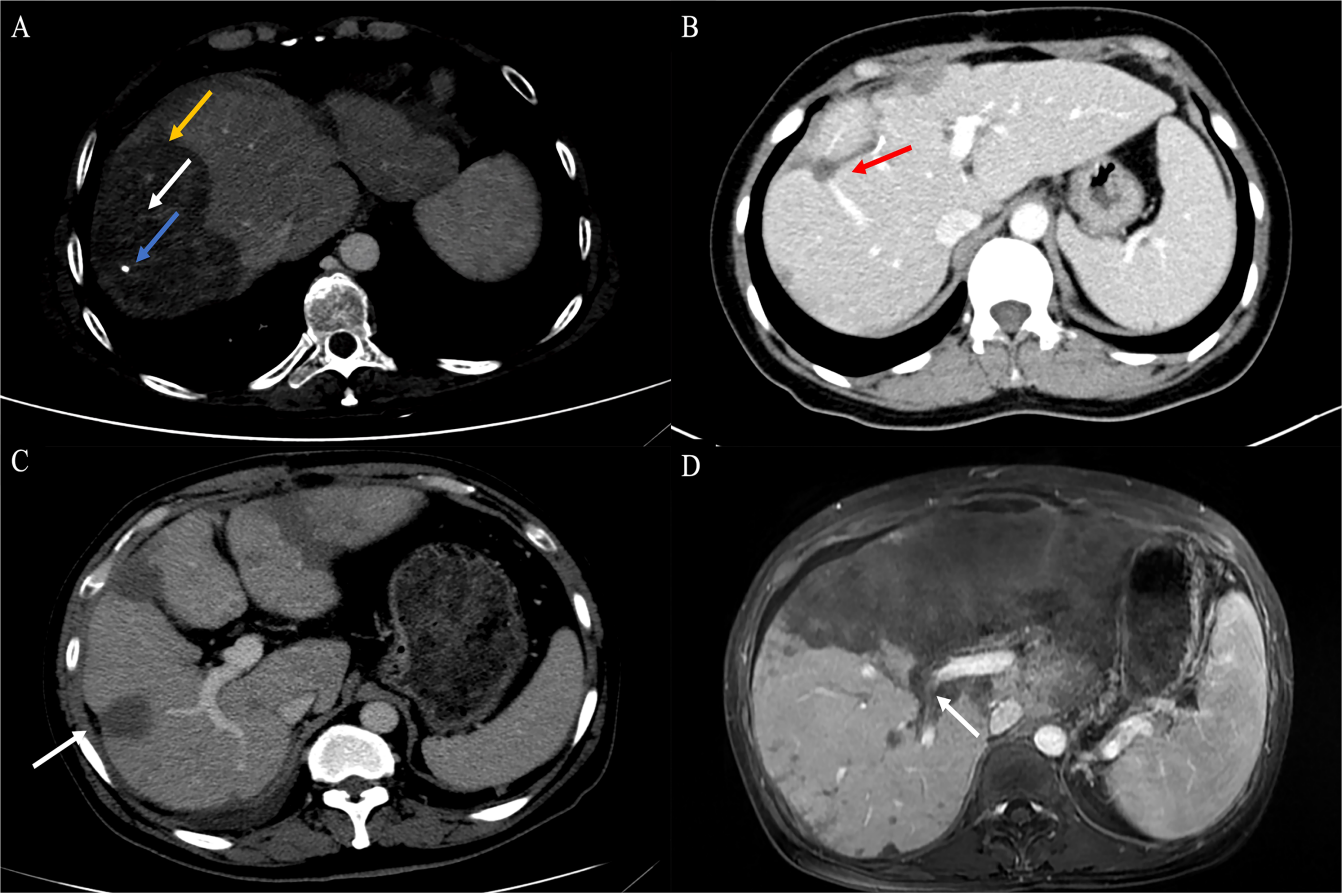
Findings of HEHE in contrast-enhanced CT. (A) Portal phase image contrast-enhanced CT demonstrated a large peripherally located lesion with calcification (blue arrow). Although the lesion was predominantly hypodense, there was a subtle non-enhancing central core (white arrow), surrounded by minimally enhancing peripheral layer (orange arrow). (B) The peripheral lesion demonstrated abrupt “cutoff” of a tributary of the RHV at the edge of a well-defined hypodense area; an example of the lollipop sign (red arrow). (C) Image of contrast-enhanced CT showed numerous variable-size hypodense nodules with hepatic capsular retraction (white arrow). (D) Contrast-enhanced CT showed diffuse coalescing hypoattenuating lesions; RPV and right posterior PV have been seen passing through the middle of the lesions (white arrow). CT, computed tomography; RHV, right hepatic vein; RPV, right branch portal vein; PV, portal vein.
